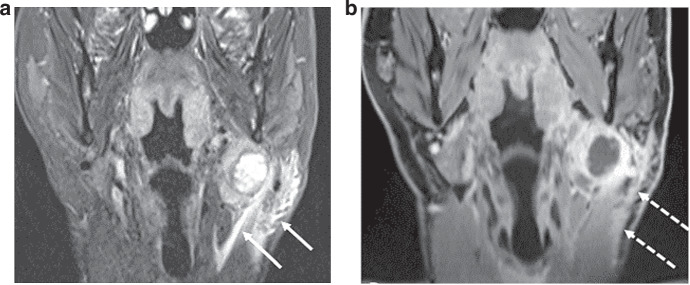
Iatrogenic changes post core biopsy—example 2. HPV-positive OPSCC 1 day following core biopsy of a necrotic left Level II node. Images show: a Coronal STIR MRI; b Coronal FS T1+c MRI, showing extensive post-biopsy oedema and stranding in the left upper neck (arrows). Note also the platysma muscle thickening and hyperenhancement (dotted arrows).
